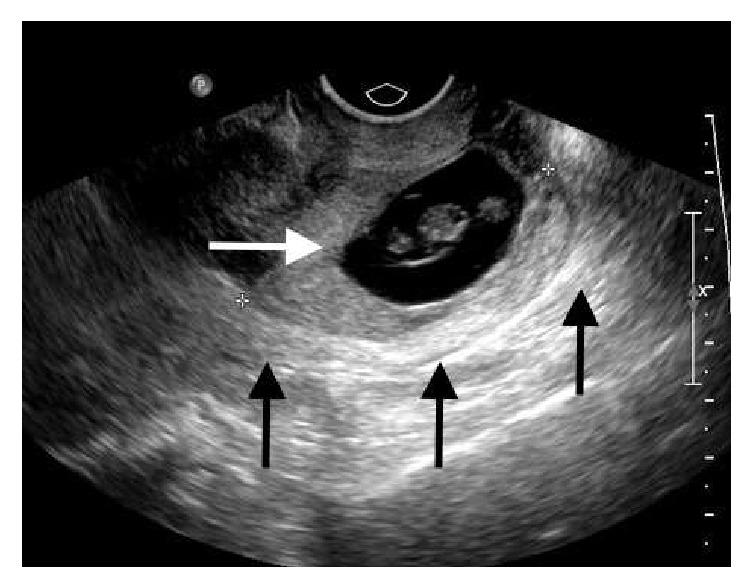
Transvaginal ultrasound showing a live ectopic pregnancy (white arrow) in the posterior wall of the cervix (black arrows). FHR 174 pbm. Estimated fetal age was 8 weeks, 1 day.
